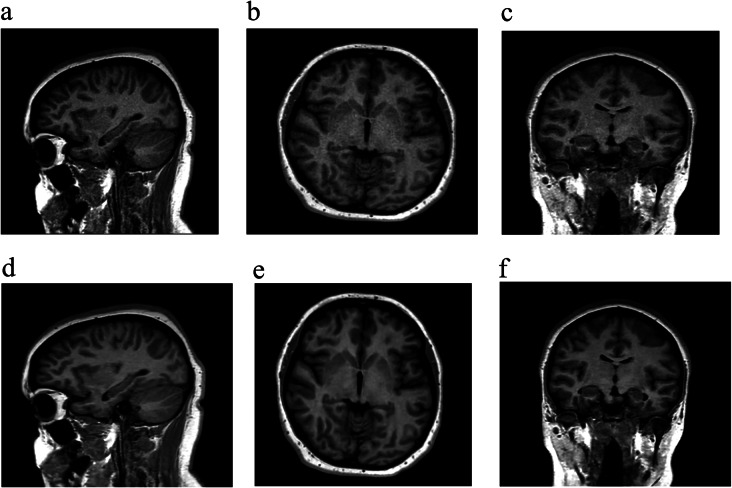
(a-f): Sagittal 3D T1-weighted SPGR CS = 5 with axial and coronal reformats in a different patient with standard (a-c) and AI (d-f) reconstruction also demonstrates improved image quality with AI
